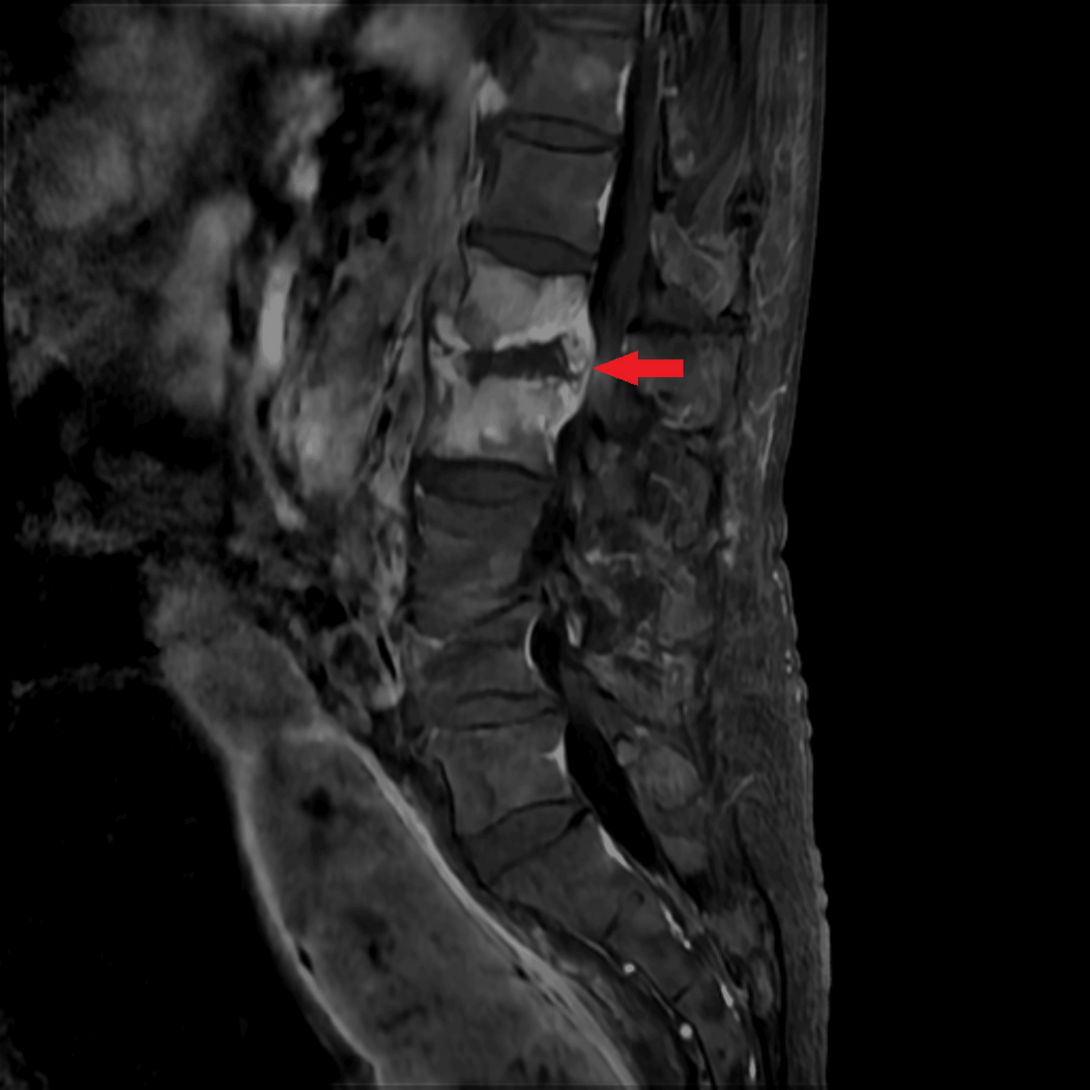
Initial enhanced magnetic resonance imaging. The L1–2 vertebral bodies show increased signal intensity, suggestive of osteomyelitis and discitis.
